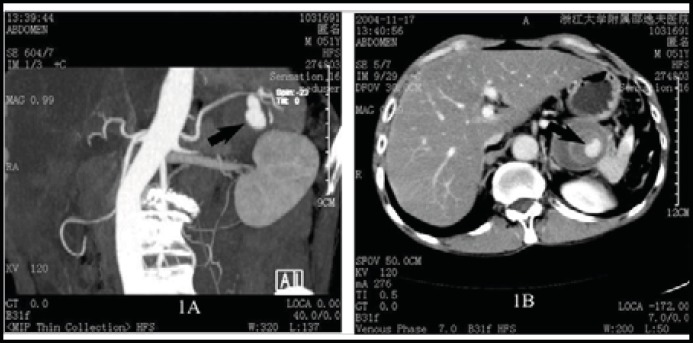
Contrast enhanced CT shows splenic artery aneurysm located near apex of left kidney (black arrow, 1A) and formation of hematoma next to the posterior wall of stomach (black arrow, 1B
